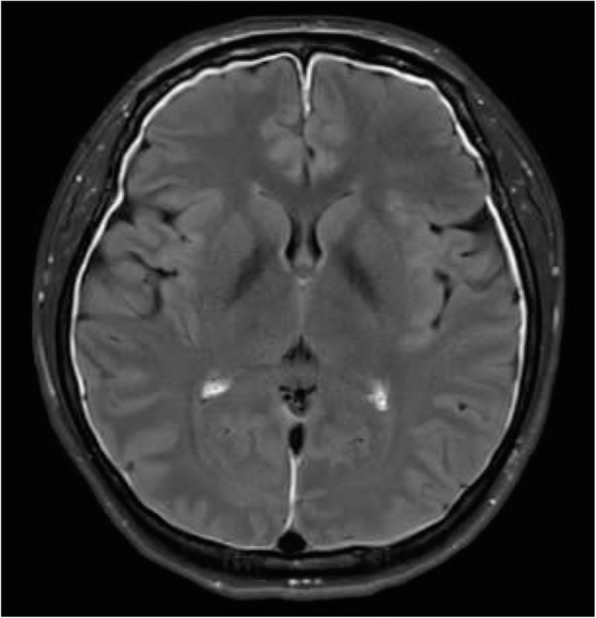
Brain MRI show diffuse pachymeningeal thickening and enhancement
